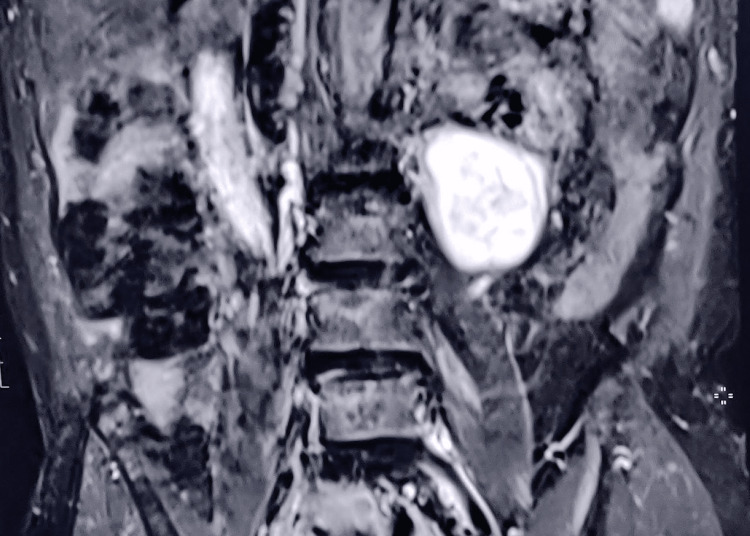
Magnetic resonance imaging of the lumbar coronal section showing a mass lesion in the left paravertebral region.
